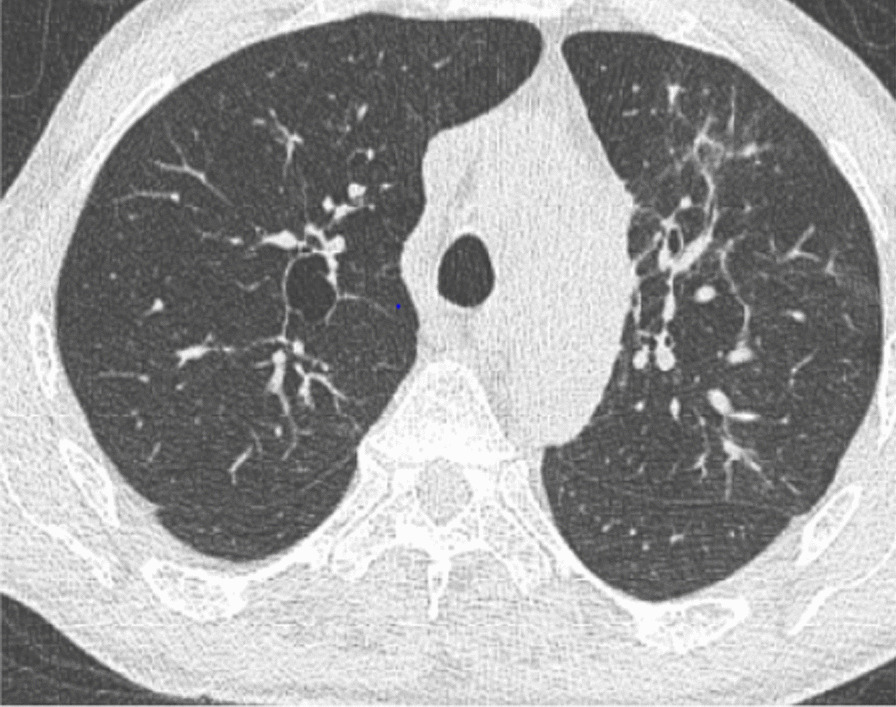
Thoracic CT scan (performed after the second bolus of cyclophosphamide) showed radiological improvement of excavated nodules with the disappearance of condensation
